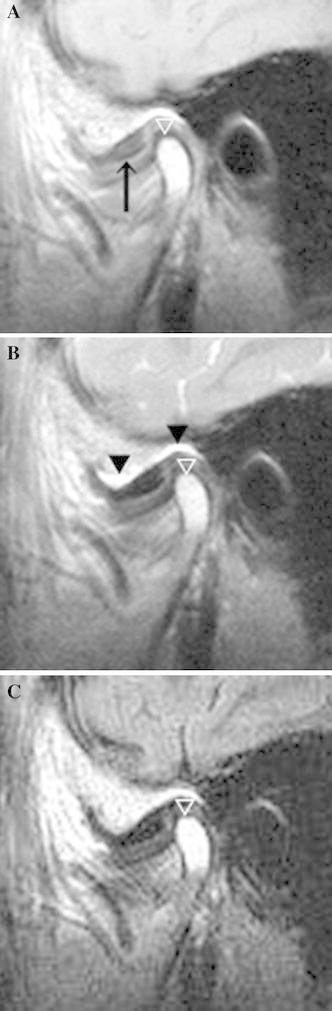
Painful TMJ with disk displacement and no reduction in a 68-year-old woman. A disk (*arrow*) was anteriorly displaced on a proton density-weighted image (a), and a large amount of joint effusion (*arrowhead*) was observed in the upper joint space on a T2-weighted image (b). The SIR was 1.90 for the bone marrow of the mandibular condyle on a FLAIR image (c). No bone marrow change was apparent on any MR images (*white arrow*) (a–c)
